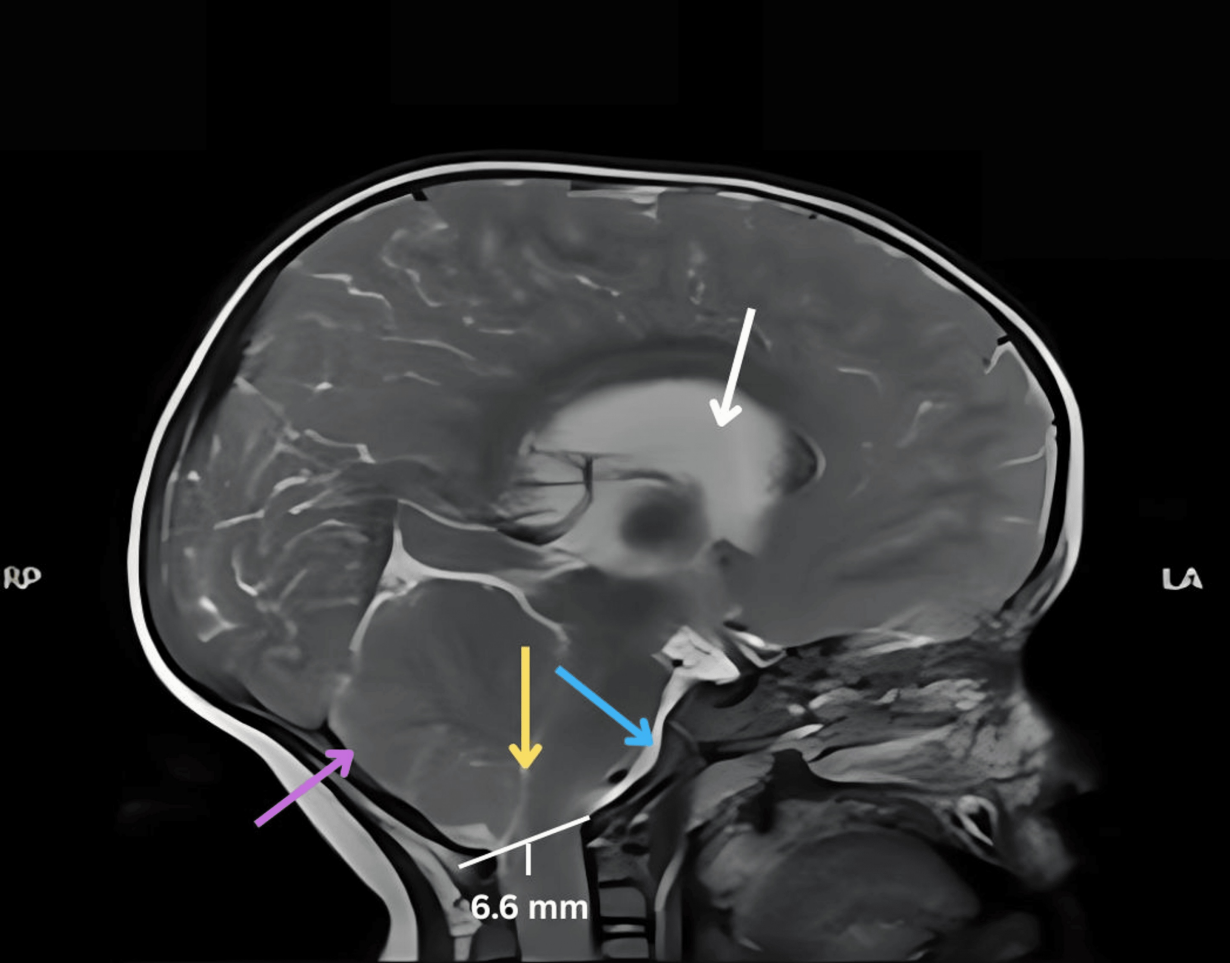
MRI sagittal T2-weighted sequence of the brain in Type 2 Chiari malformation showing supratentorial ventriculomegaly (white arrow). The bony posterior fossa appears small and shallow with a dysplastic concave clivus (blue arrow). The posterior fossa is crowded with effacement of premedullary and retrocerebellar cerebrospinal fluid spaces (purple arrow). The fourth ventricle is slit-like and obliterated (yellow arrow). Herniation of the cerebellar tonsil is seen beyond the opisthion–basion line, measuring a distance of 6.6 mm.
